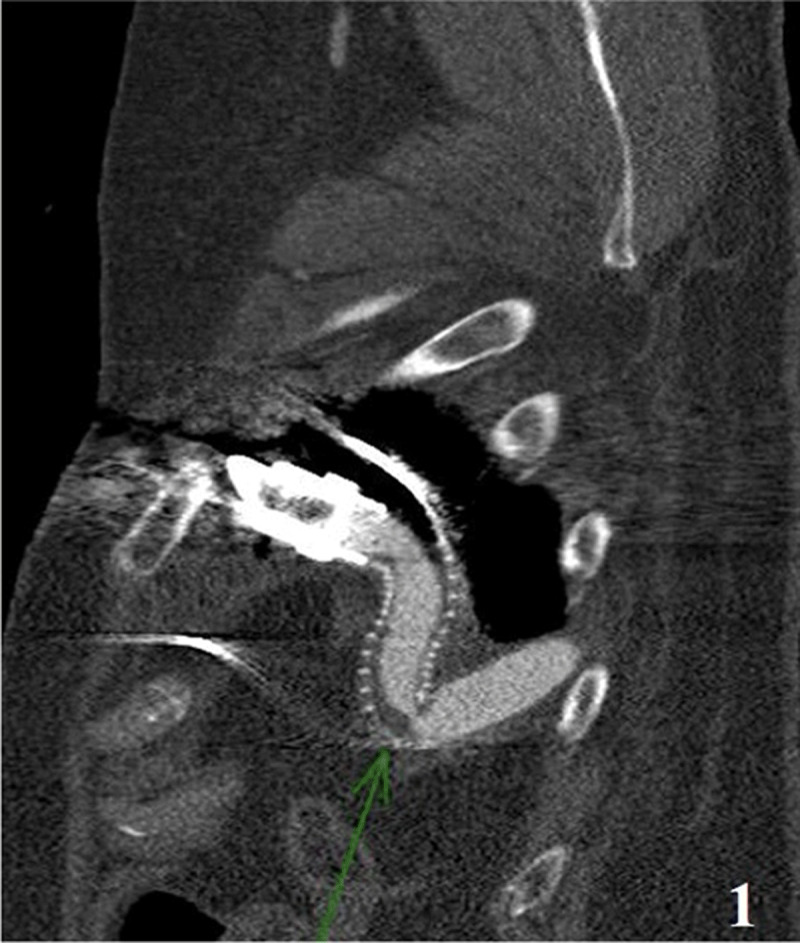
Cardiac computed tomography showing left ventricular assist device outflow graft obstruction.
